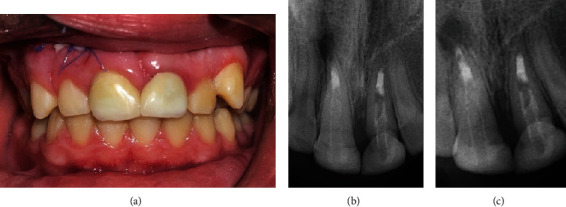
One-week postsurgery follow-up. (a) Clinical image showing healing of the surgery site. Mild gingival inflammation can be seen distal to tooth #21. PA radiographs of (b) before and (c) after surgery.
